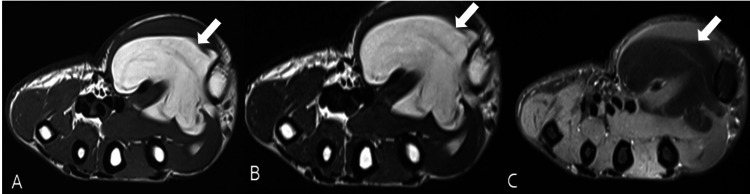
MRI of the wrist showing lipoma Axial MRI images of the wrist in a 35-year-old female with swelling in the right thenar region showing a well-defined lobulated lesion localized in the intermuscular plane deep to the thenar muscles, which appears hyperintense on T1-weighted (A) and T2-weighted (B) images and showing signal suppression on two-dimensional (2D) fast spin-echo (FSE) fat-suppressed proton density image (C). Diagnosis: lipoma.
